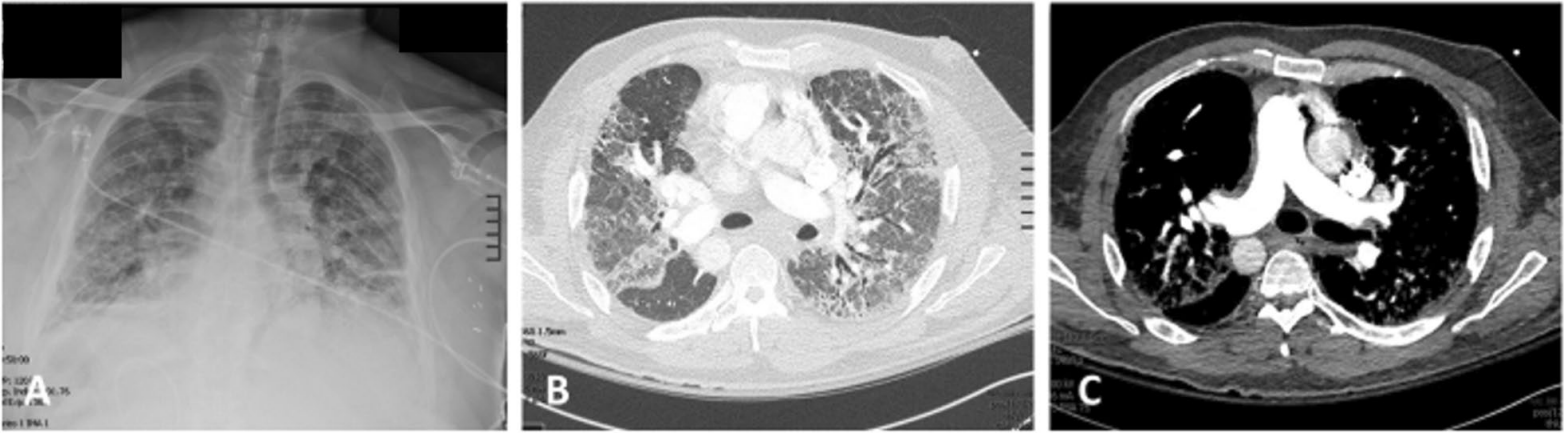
Chest X-ray and computed tomographic images (A–C), obtained prior to the lung transplantation, showing evidence of interstitial lung disease changes with situs inversus totalis
